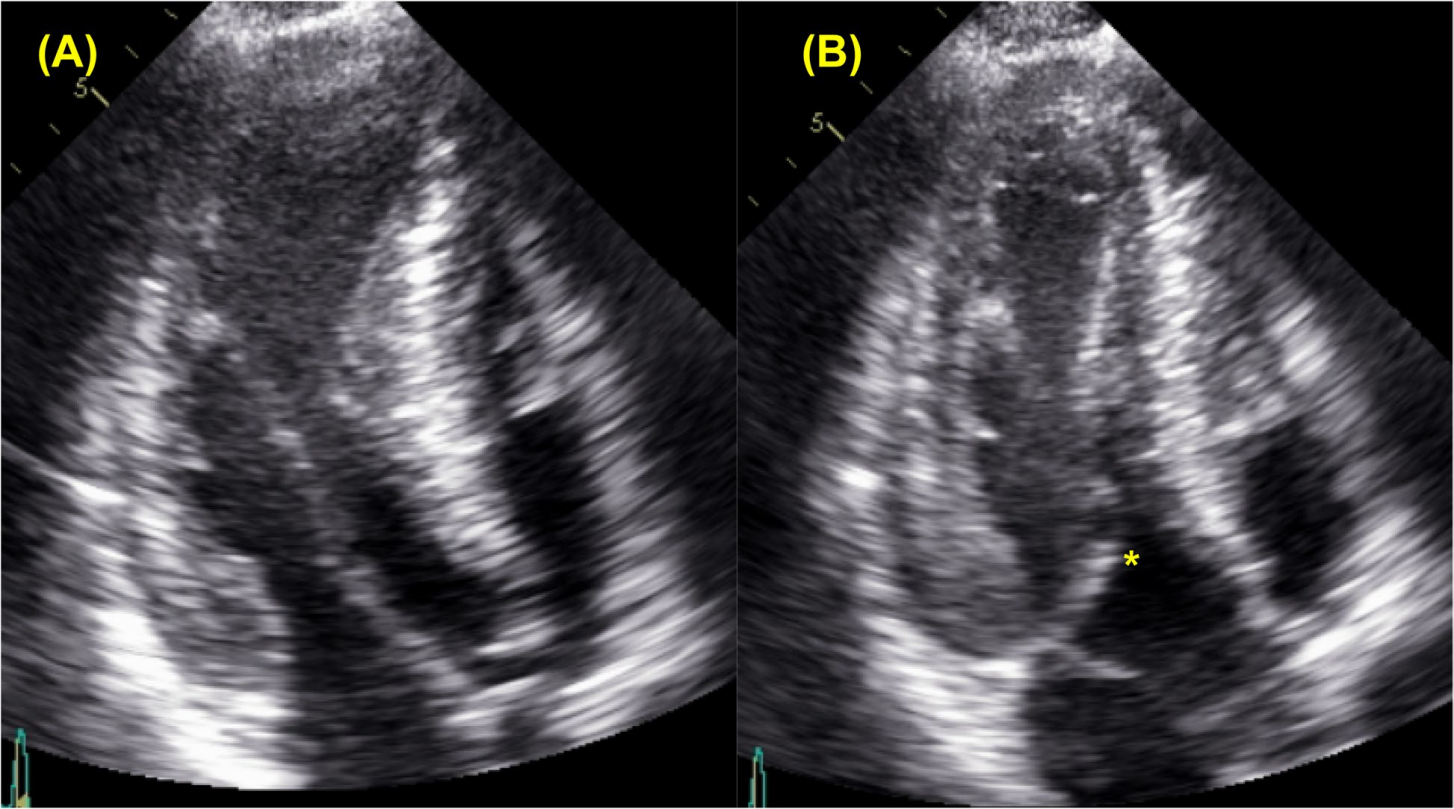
Transthoracic echocardiography of patients with obstructive hypertrophic cardiomyopathy. Apical three-chamber view at (A) end-diastole and B during systole demonstrate systolic anterior motion of the mitral valve (asterisk)
